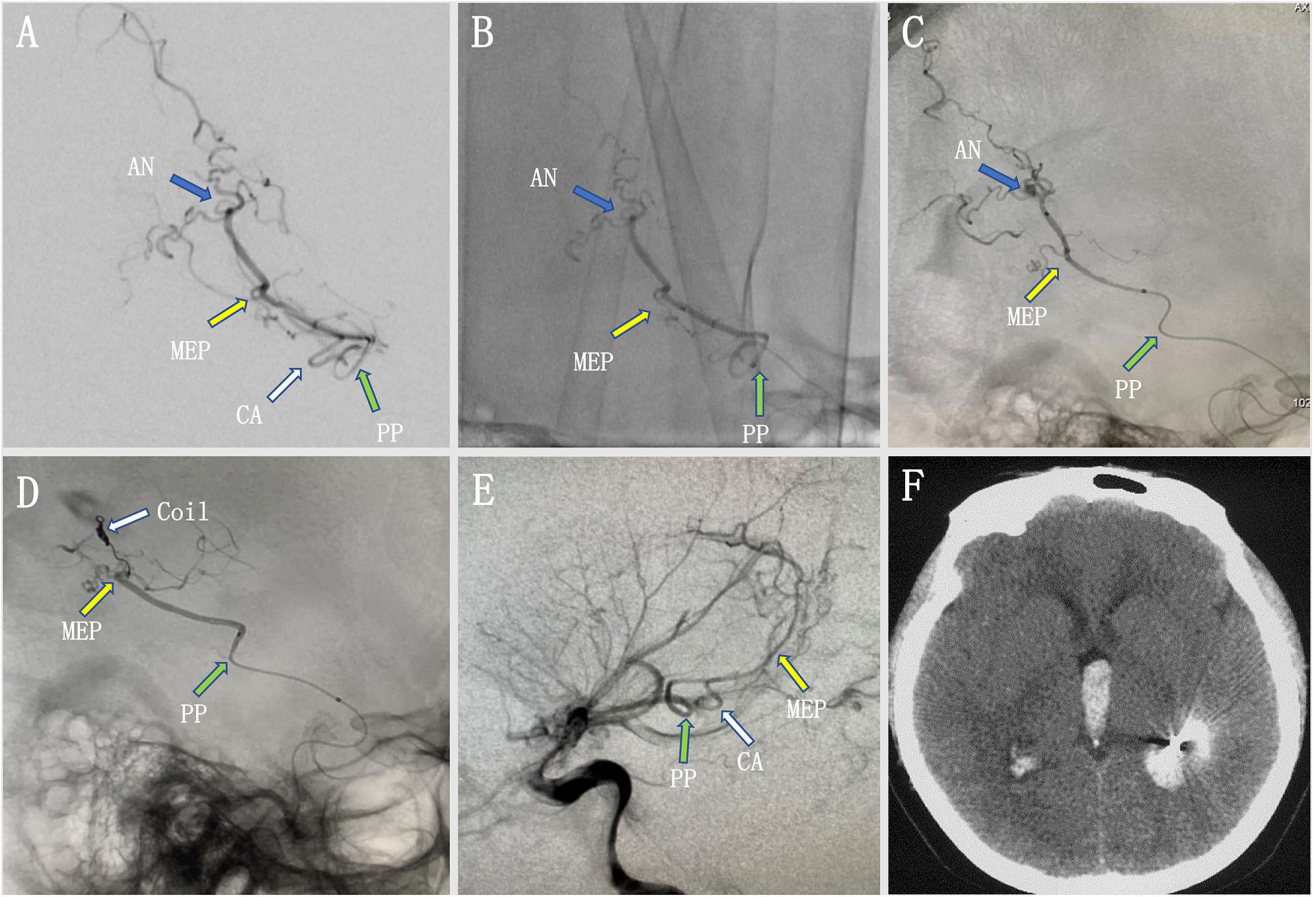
(A–C) Ultra-selective digital subtracted angiography (DSA) at the plexal point (PP) (A) in the operation showed a large capsulothalamic artery arising at the PP. The ultra-selective DSA at the most external point (MEP) (B) showed two other branches arising at the MEP of the anterior choroidal artery (AChoA). The ultra-selective DSA (C) showed the microcatheter tip had crossed the MEP and another branch arising near the distal AChoA aneurysm. Therefore, we decided to embolize the aneurysm and the distal AChoA by coils. (D,E) Ultra-selective DSA (D) showed that the distal AChoA aneurysm and the AChoA beyond the MEP were embolized by coils, and DSA of the left internal carotid artery (ICA) (E) showed that there was no retrograde filling of the aneurysm by collaterals and that the ipsilateral capsulothalamic artery had been retained. (F) Postoperative head computed tomography (CT) showed that the AChoA aneurysm was embolized beyond the MEP with coils, and the intraventricular hemorrhage had disappeared.
